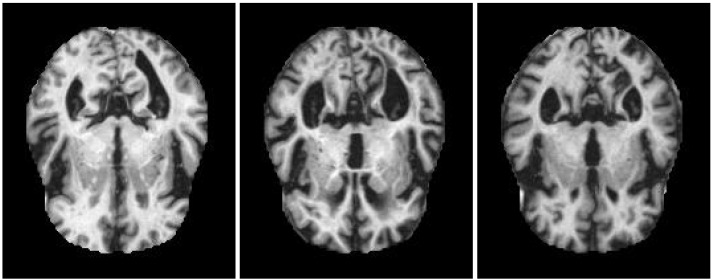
Example of items from the three classes. Left pane: *non-demented*. Center pane: *mild demented*. Right pane: *moderate demented*. MRI images contained in this figure are taken from the original Alzheimer’s data set [53] available on Kaggle.
